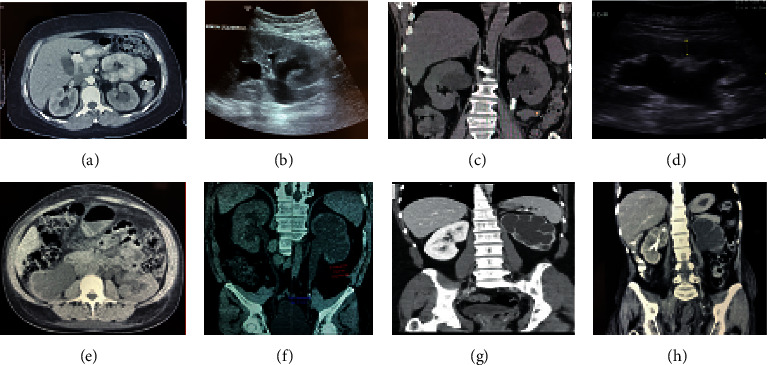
Hydronephrosis grades in ultrasonogram and tomography images. Grade I, slight blunting of calyceal fornices; Grade II, blunting and enlargement of calyceal fornices but easily seen shadow of papillae; Grade III, rounding of calices with obliteration of papillae; Grade IV, extreme calyceal ballooning.
